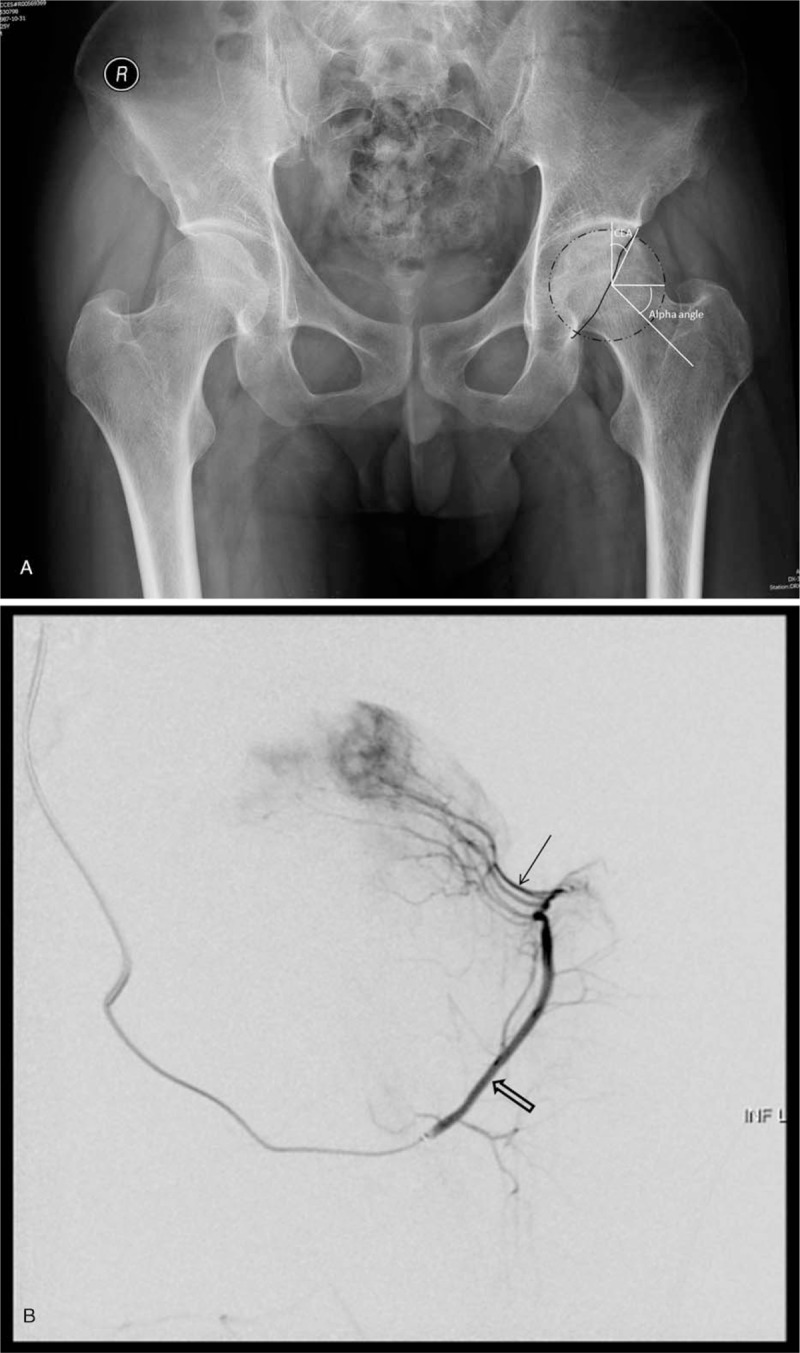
(A) X-ray image of a 35-year-old male patient. (B) DSA examination of the same patient in (A).
